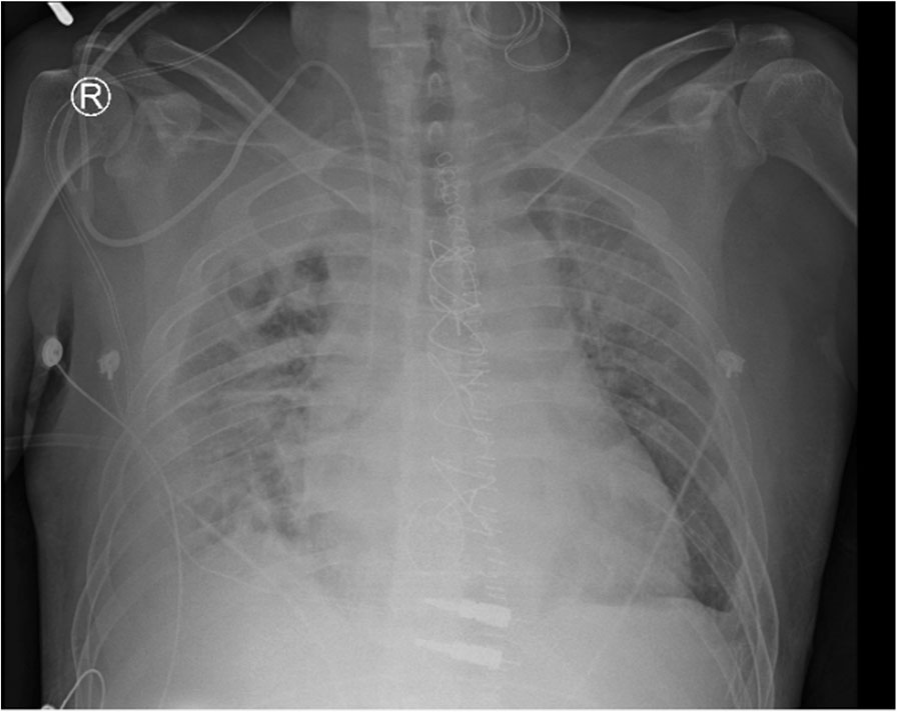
Follow-up chest X-ray 14 days after tricuspid valve replacement shows loculated bilateral pleural effusions with perihilar and lower lung haziness infiltrates
